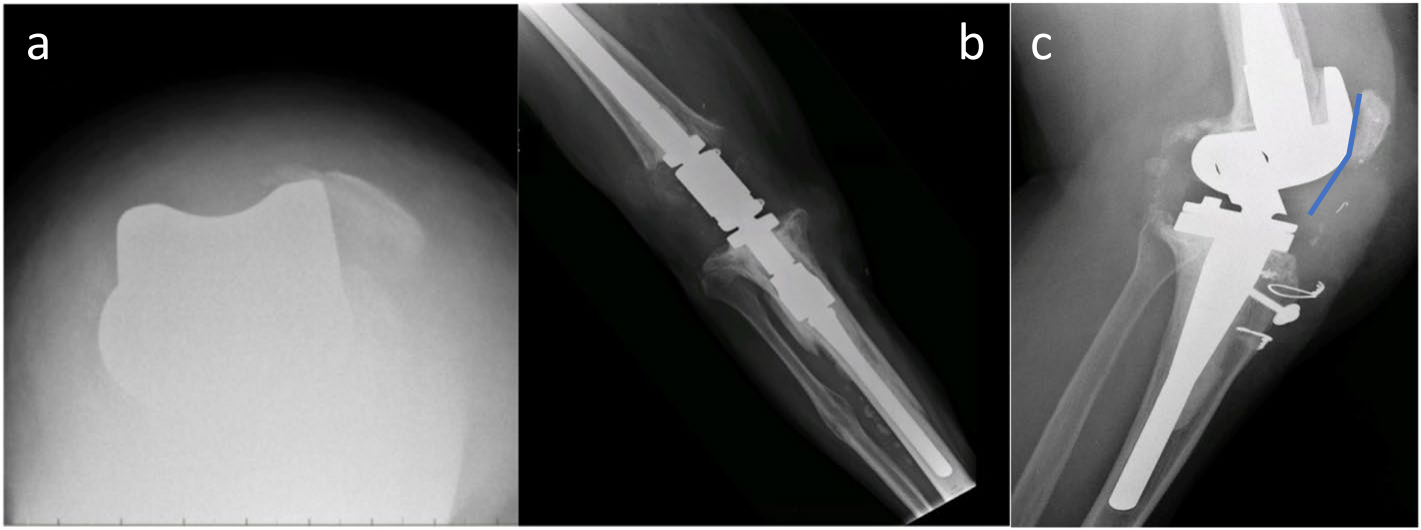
a. X-ray of patellar dislocation b. X-ray of knee arthrodesis c. Caton-Deschamps index
